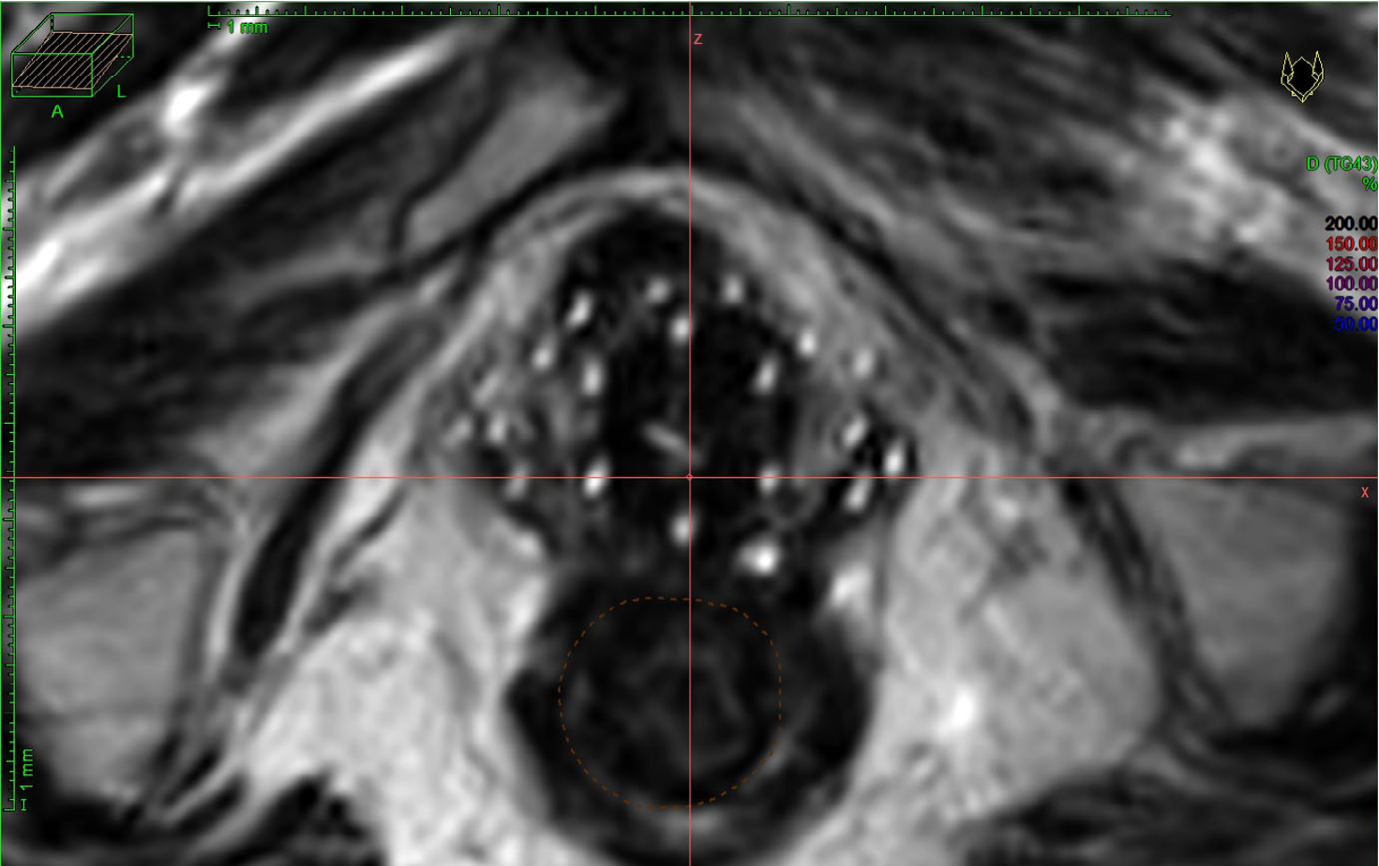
Catheters visualized by MRI line markers in T2 weighted MRI axial scan image. MRI, magnetic resonance imaging.
